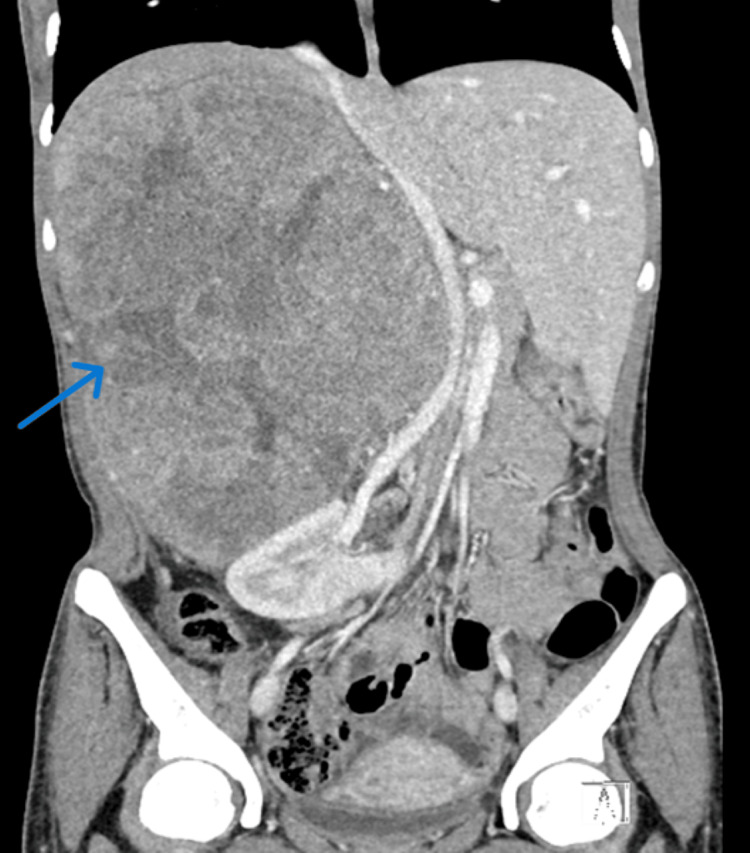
Coronal view of the CT scan of the abdomen showing the proximity of the mass to the IVC and displacement of the IVC, liver, and right kidney IVC: inferior vena cava
